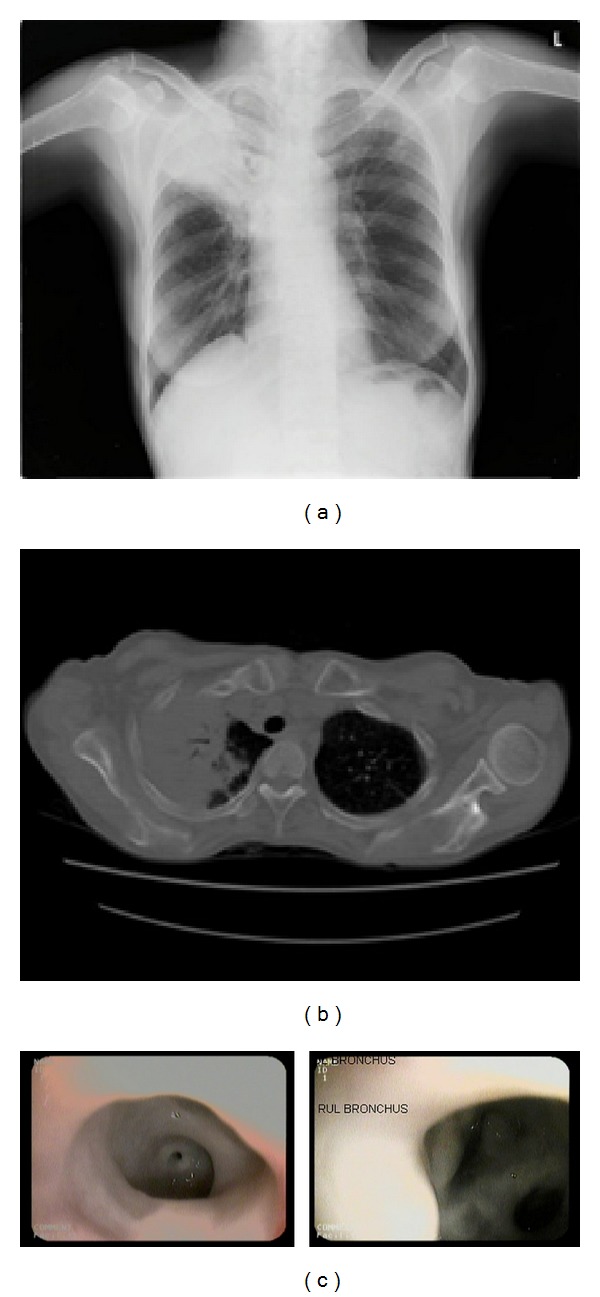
(a) Chest X ray at presentation showing right upper lobe homogenous opacity. (b) CT chest showing dense peripheral consolidation of the right upper lobe. (c) Bronchoscopic picture of right upper lobe showing cheesy pseudomembrane lining the right upper lobe bronchial segments.
